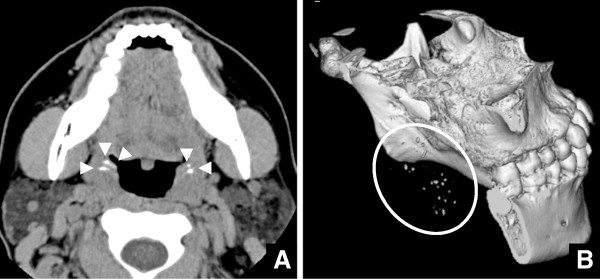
CT images of a 31-year-old female with 28 tonsilloliths. A) Axial CT images at the palatine tonsillar level. Twenty-eight tonsilloliths (arrowheads) are seen as high-density, dot-like structures. B) Three-dimensional reconstruction images of CT. Twenty-eight tonsilloliths within a circle are shown as high density dot-like structures.
